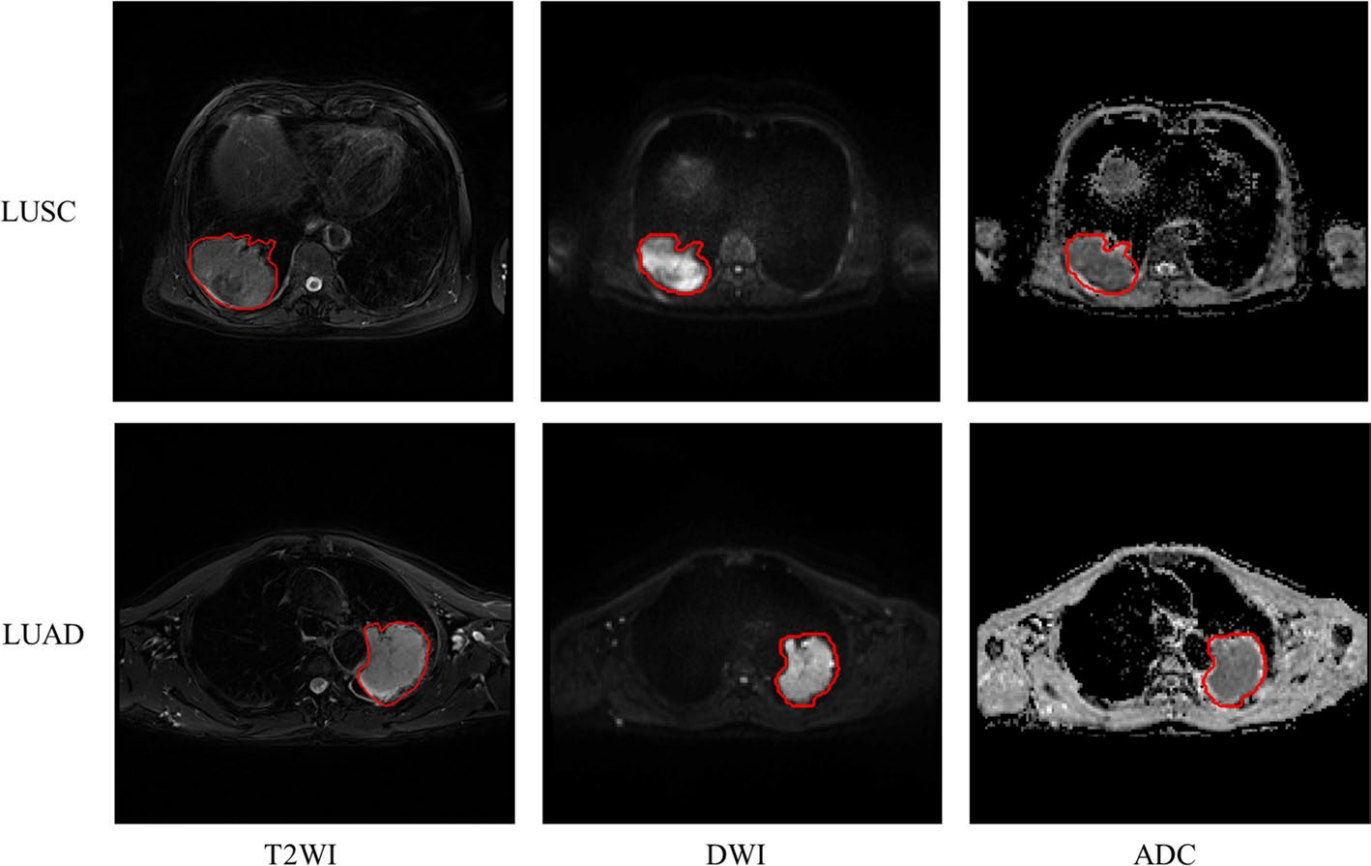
Examples of the delineated lung squamous cell carcinoma (LUSC) and lung adenocarcinoma (LUAD) on the multimodal MRI data
